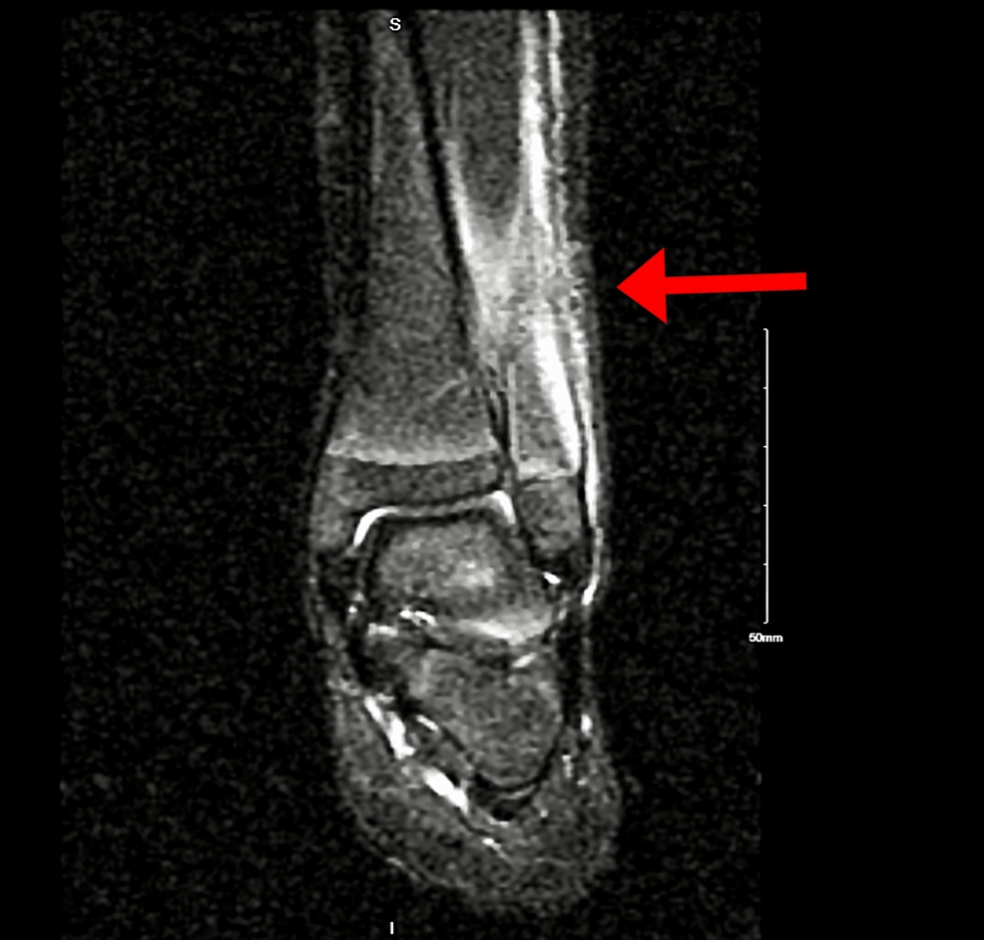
MRI of the left lower extremity showing subperiosteal collection (red arrow) and changes consistent with osteomyelitis
